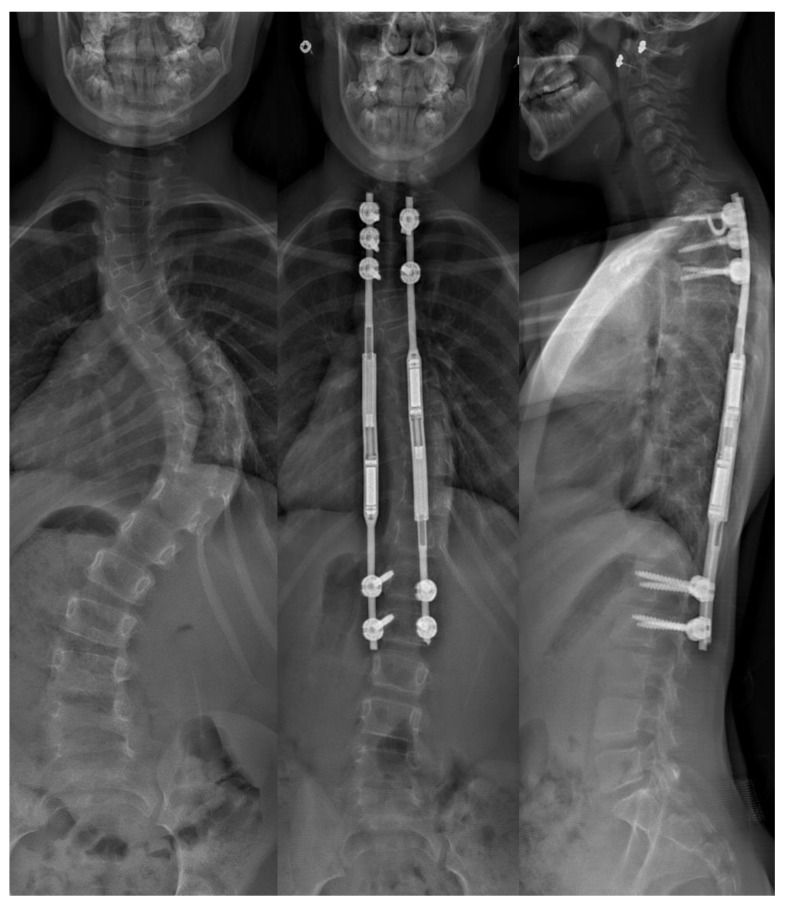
Left: preoperative of large magnitude scoliosis, Center: postoperative anterior-posterior radiograph, Right: lateral radiographs following the application of two magnetic growing rods with significant improvement of the curvature.
